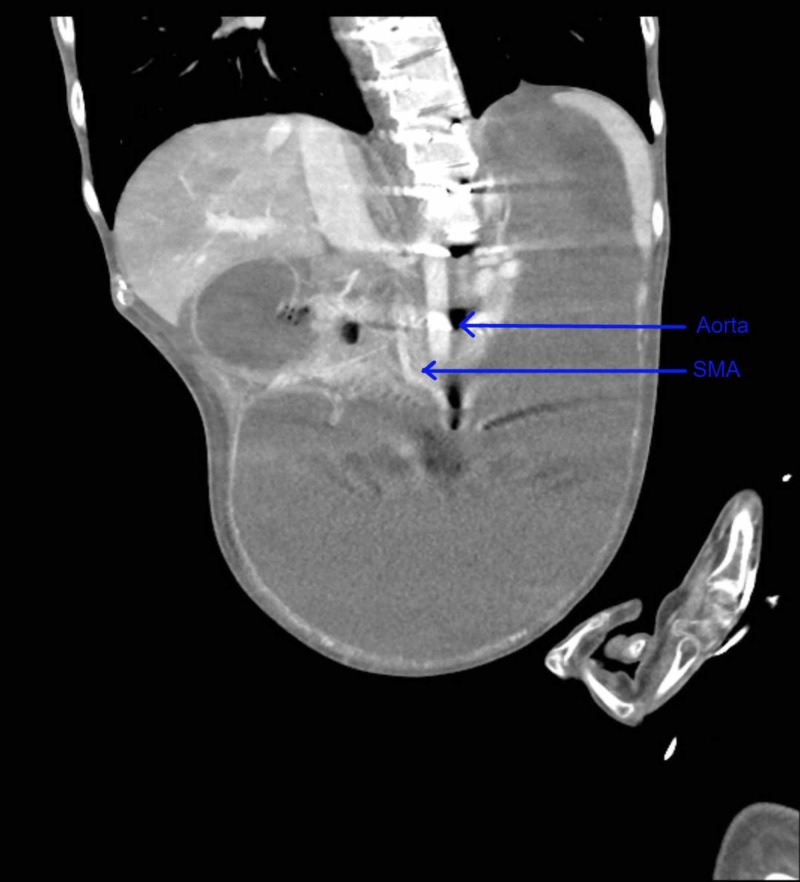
Computed tomography (CT) scan of the abdomen (coronal section) with contrast showing massively distended stomach extending into the pelvis SMA: superior mesenteric artery.
